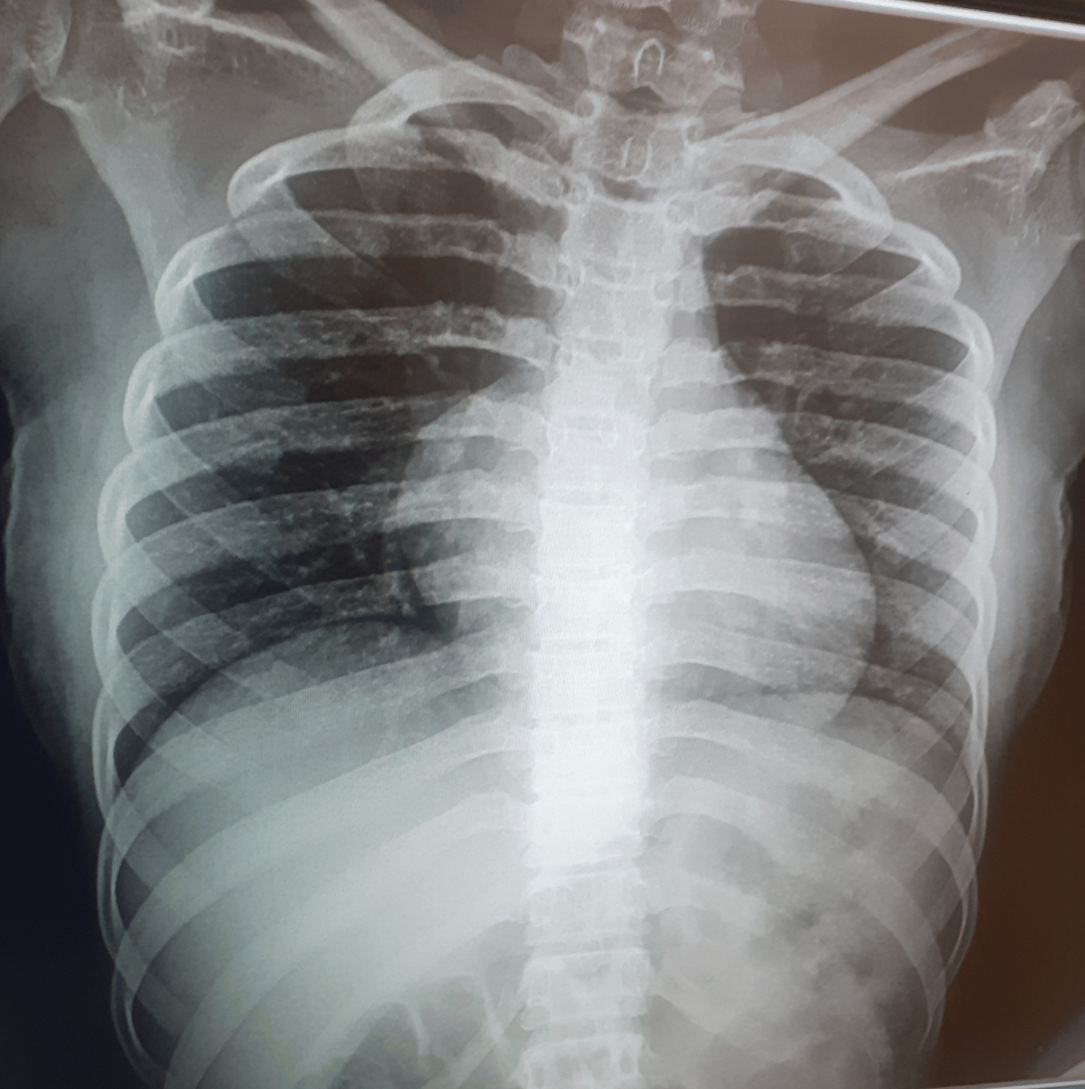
Chest X-ray showing normal findings.
